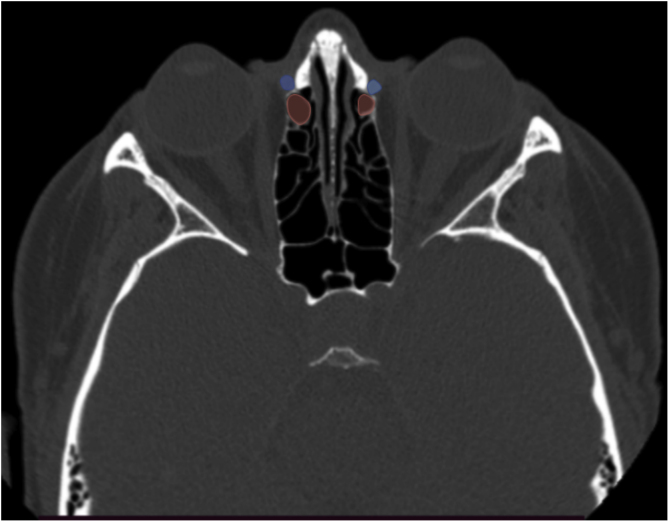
CT scan (axial slice) showing agger nasi cell (red) located posterior to the lacrimal sac (blue) bilateral.
